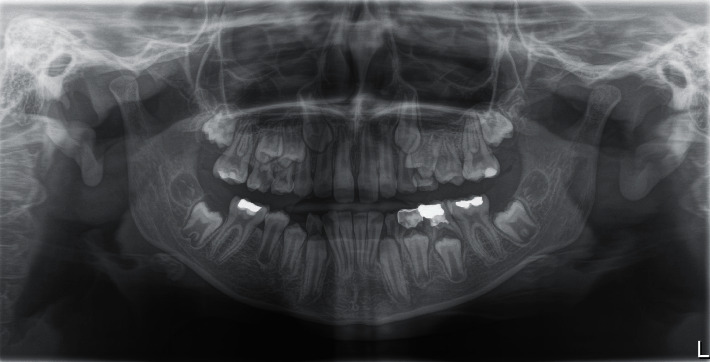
OPG X-ray taken during routine check-up of the patient, two years prior to the diagnosis of the cyst, showing no signs of pathology in left premolar region of the mandible at the time.
